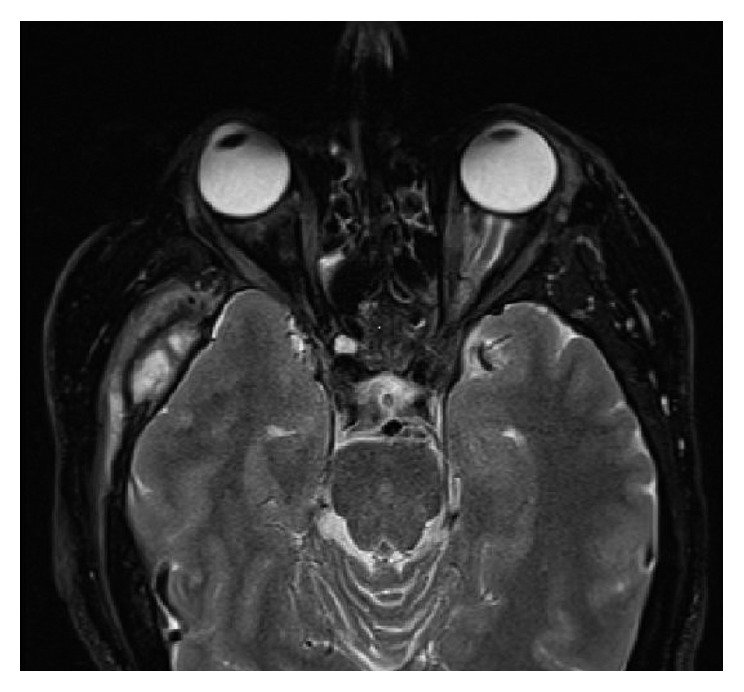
MRI brain showing a right orbital mass and a left mastoid mass.
